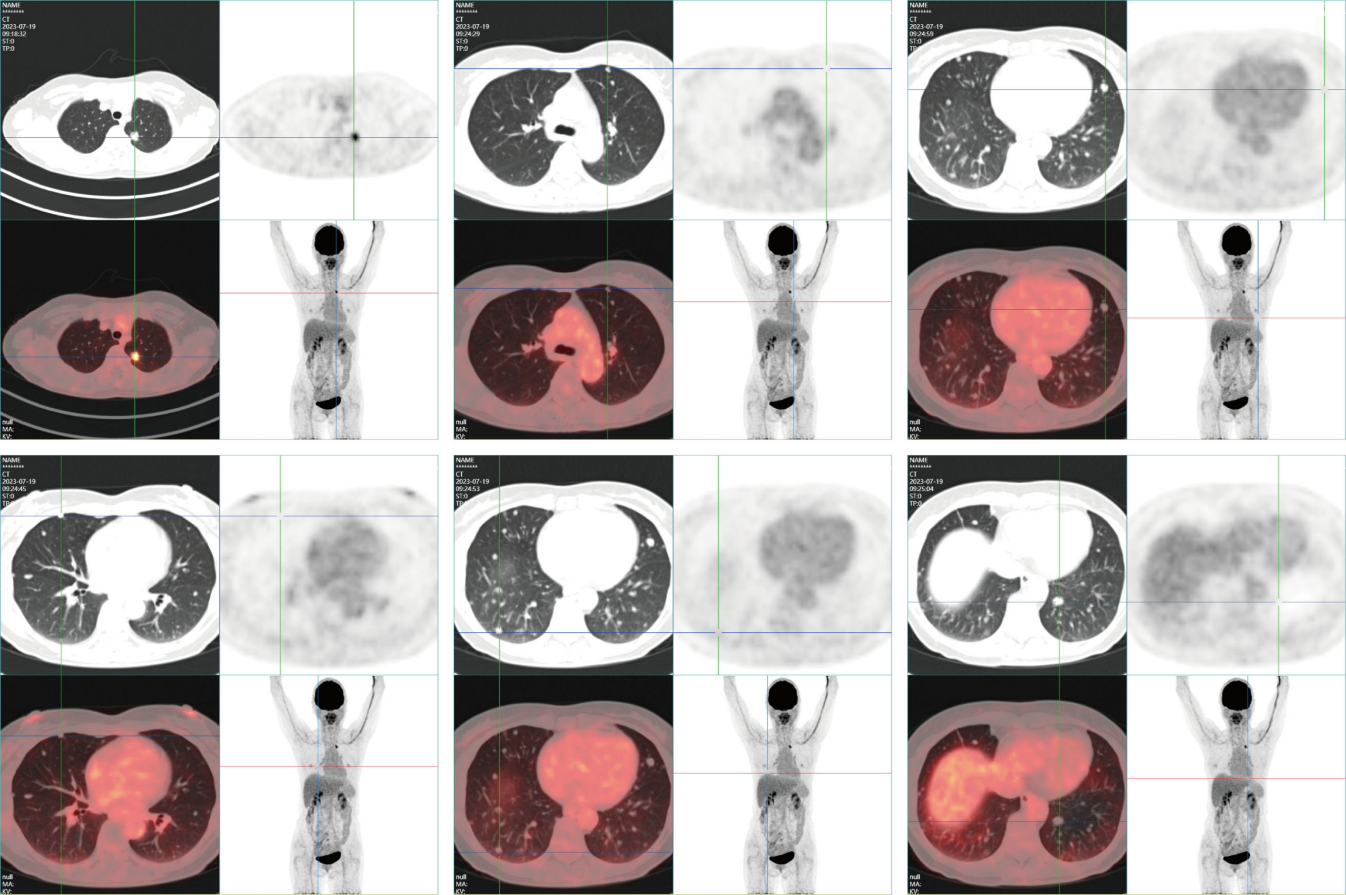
PET-CT reveals an irregular nodule subpleurally in the apicoposterior segment of the left upper lobe, measuring approximately 1.4x1.3 cm. The nodule, adorned with minute spiculations, exerts traction on the adjacent pleura and exhibits abnormal radiotracer accumulation, with an SUVmax of 7.1. Multiple variably sized, quasicircular nodules are identified in both lungs, with diameters ranging from approximately 0.2-1.0 cm; these do not exhibit abnormal radiotracer concentrations. No additional abnormal solid changes or radiotracer accumulations are observed. No evident enlargement or abnormal radiotracer uptake was identified in the mediastinal lymph nodes.
